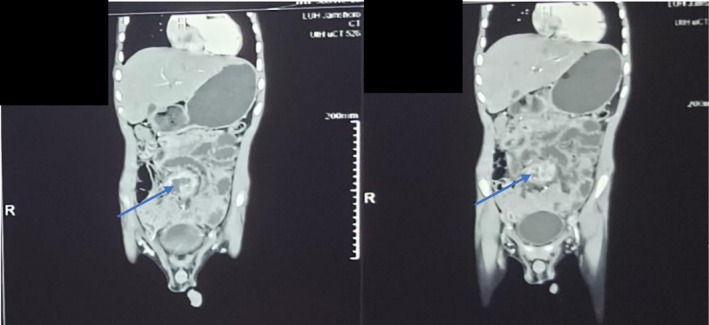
Coronal contrast‐enhanced CT scan of the abdomen demonstrating a large, complex inflammatory mass measuring 3.7 × 4.6 × 7.1 cm in the anterior abdominal wall. The mass is characterized by a thick, peripherally enhancing wall and a central necrotic component, consistent with an abscess within a urachal phlegmon (Arrow).
